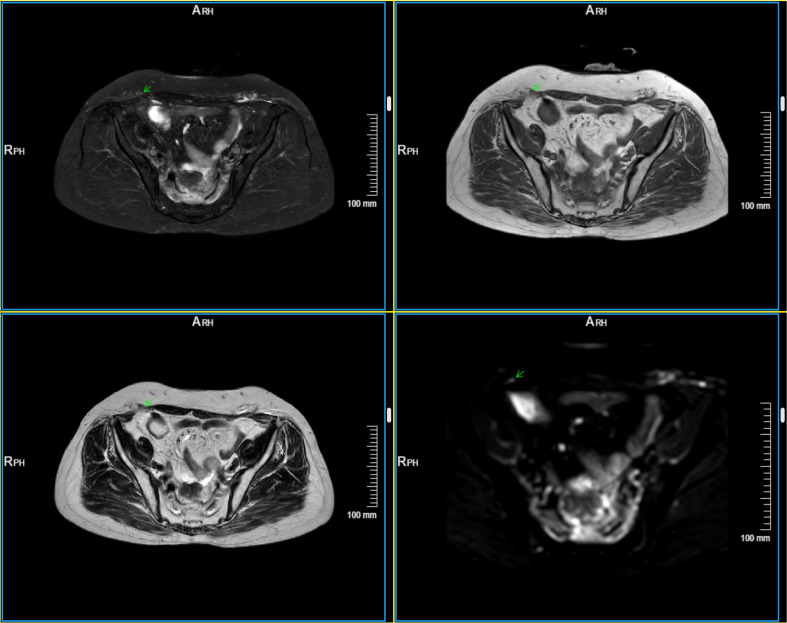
Contrast-enhanced MRI scan in May 2024. T2-weighted fat-saturated image (left upper) shows a hyperintense peritoneal nodule in the anterior abdominal wall (arrow). The lesion appears isointense to hypointense on axial T1-weighted image (right upper) with definite localization. Conventional T2-weighted image (left lower) is applied for comparison to exclude artifacts. Marked diffusion restriction is observed on diffusion-weighted imaging (DWI) (right lower).
